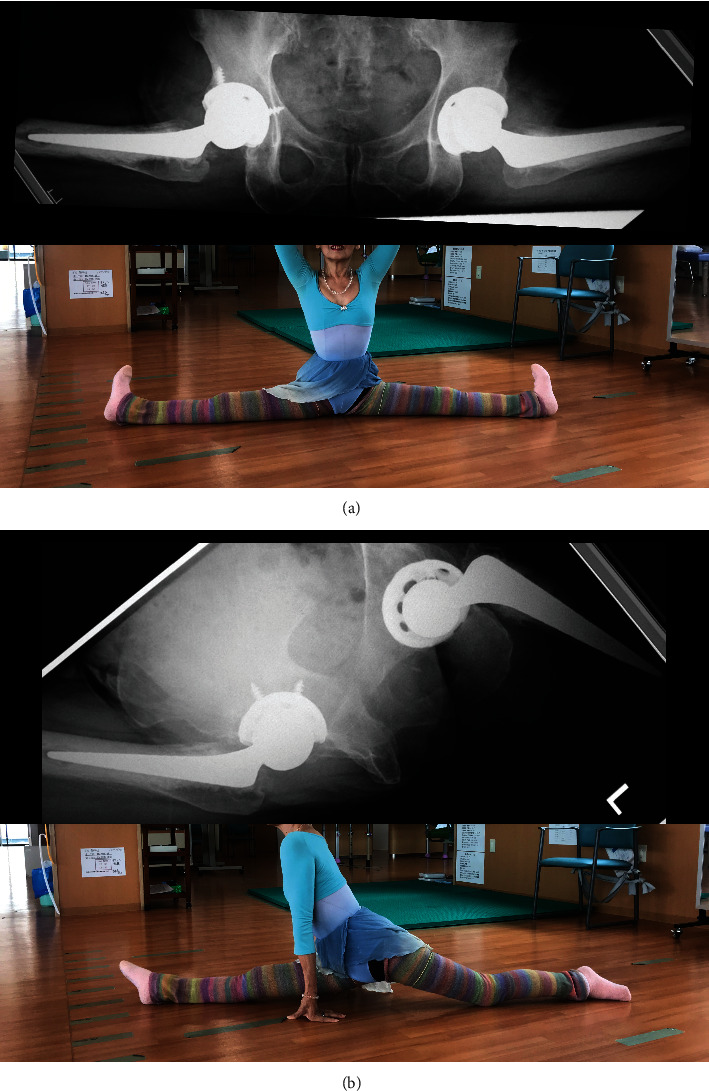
Postoperative radiograph with two types of characteristic postures in ballet. The patient was demonstrating the split position. These views were obtained with cassettes by getting as close as possible to the patient's back during the actual postures. The radiograph did not show any characteristics of bony impingement, implant-implant impingement, or subluxation of the femoral head: (a) split position; (b) left split position.
